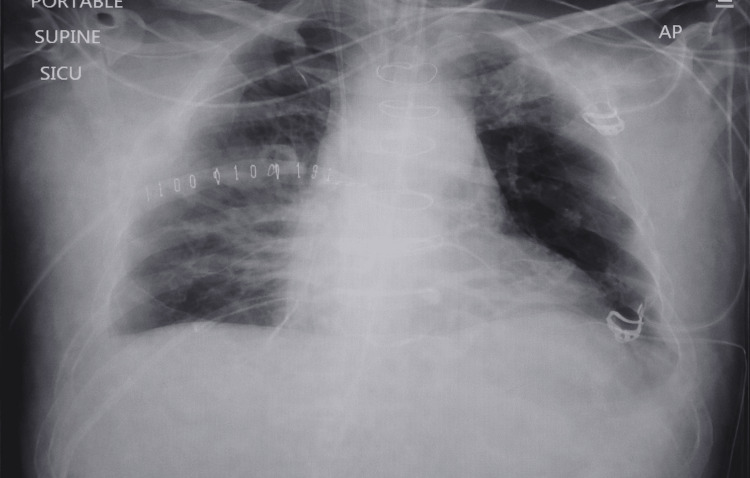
Chest X-ray of the patient post-operative demonstrating improvement of the lung infiltration
